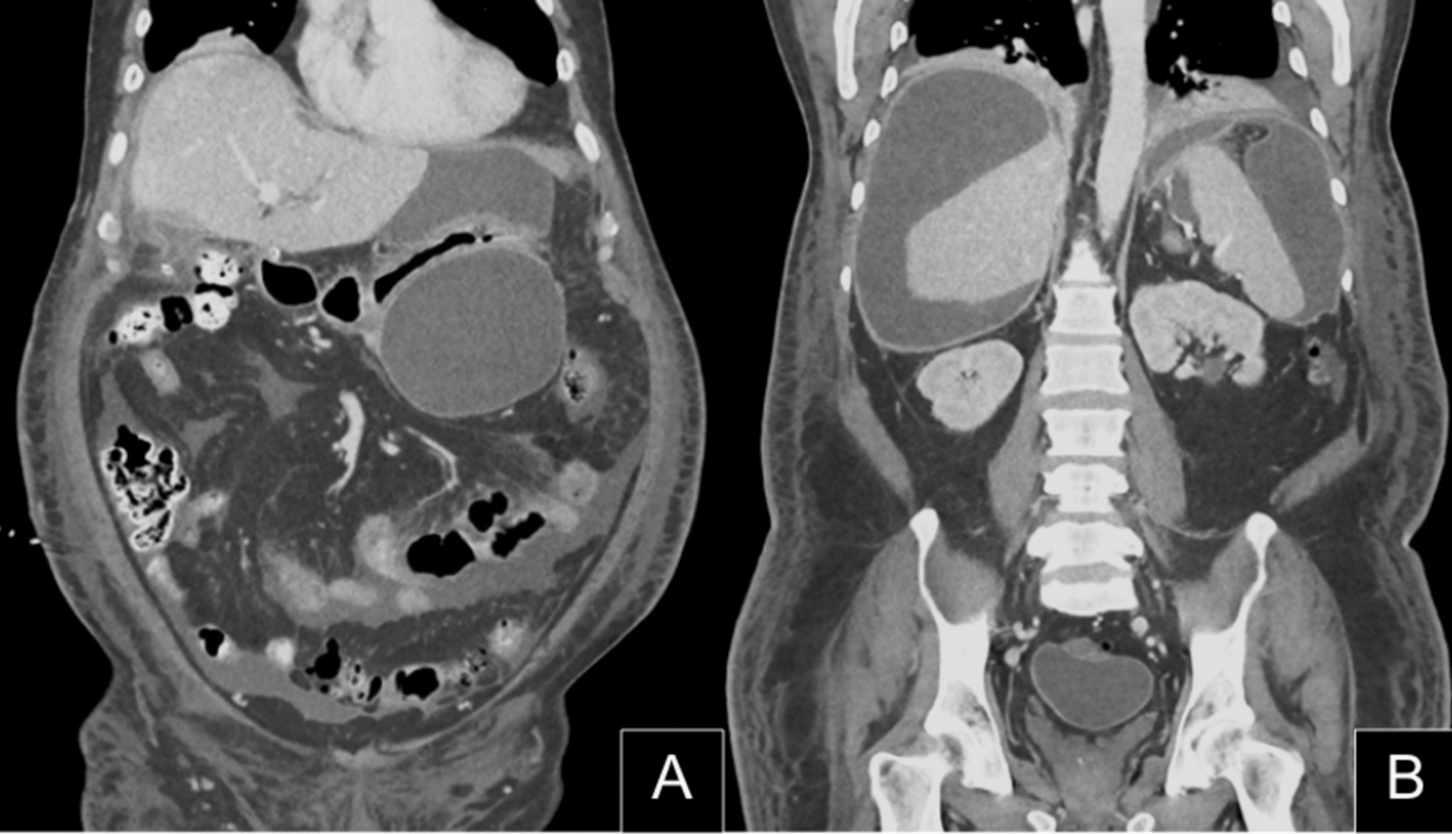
Representative coronal images from the initial CT scan (A) Free fluid and several loculated fluid collections are noted around the stomach. (B) Free fluid and several loculated fluid collections are noted around the liver and spleen as well. Small-volume reactive bilateral pleural effusions are also seen as well as pneumobilia.
